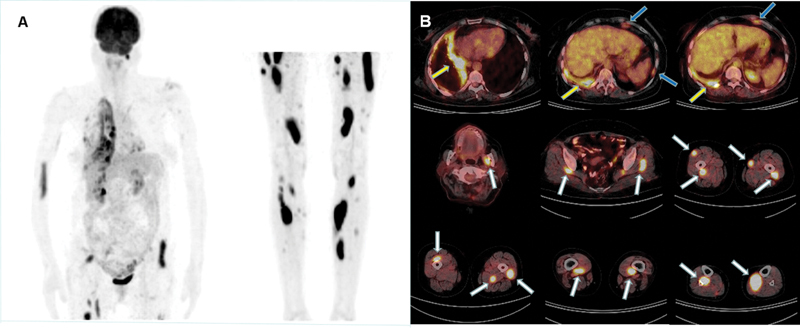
Increased fluorodeoxyglucose (FDG) uptake in the recurrent right pleural tumor (
